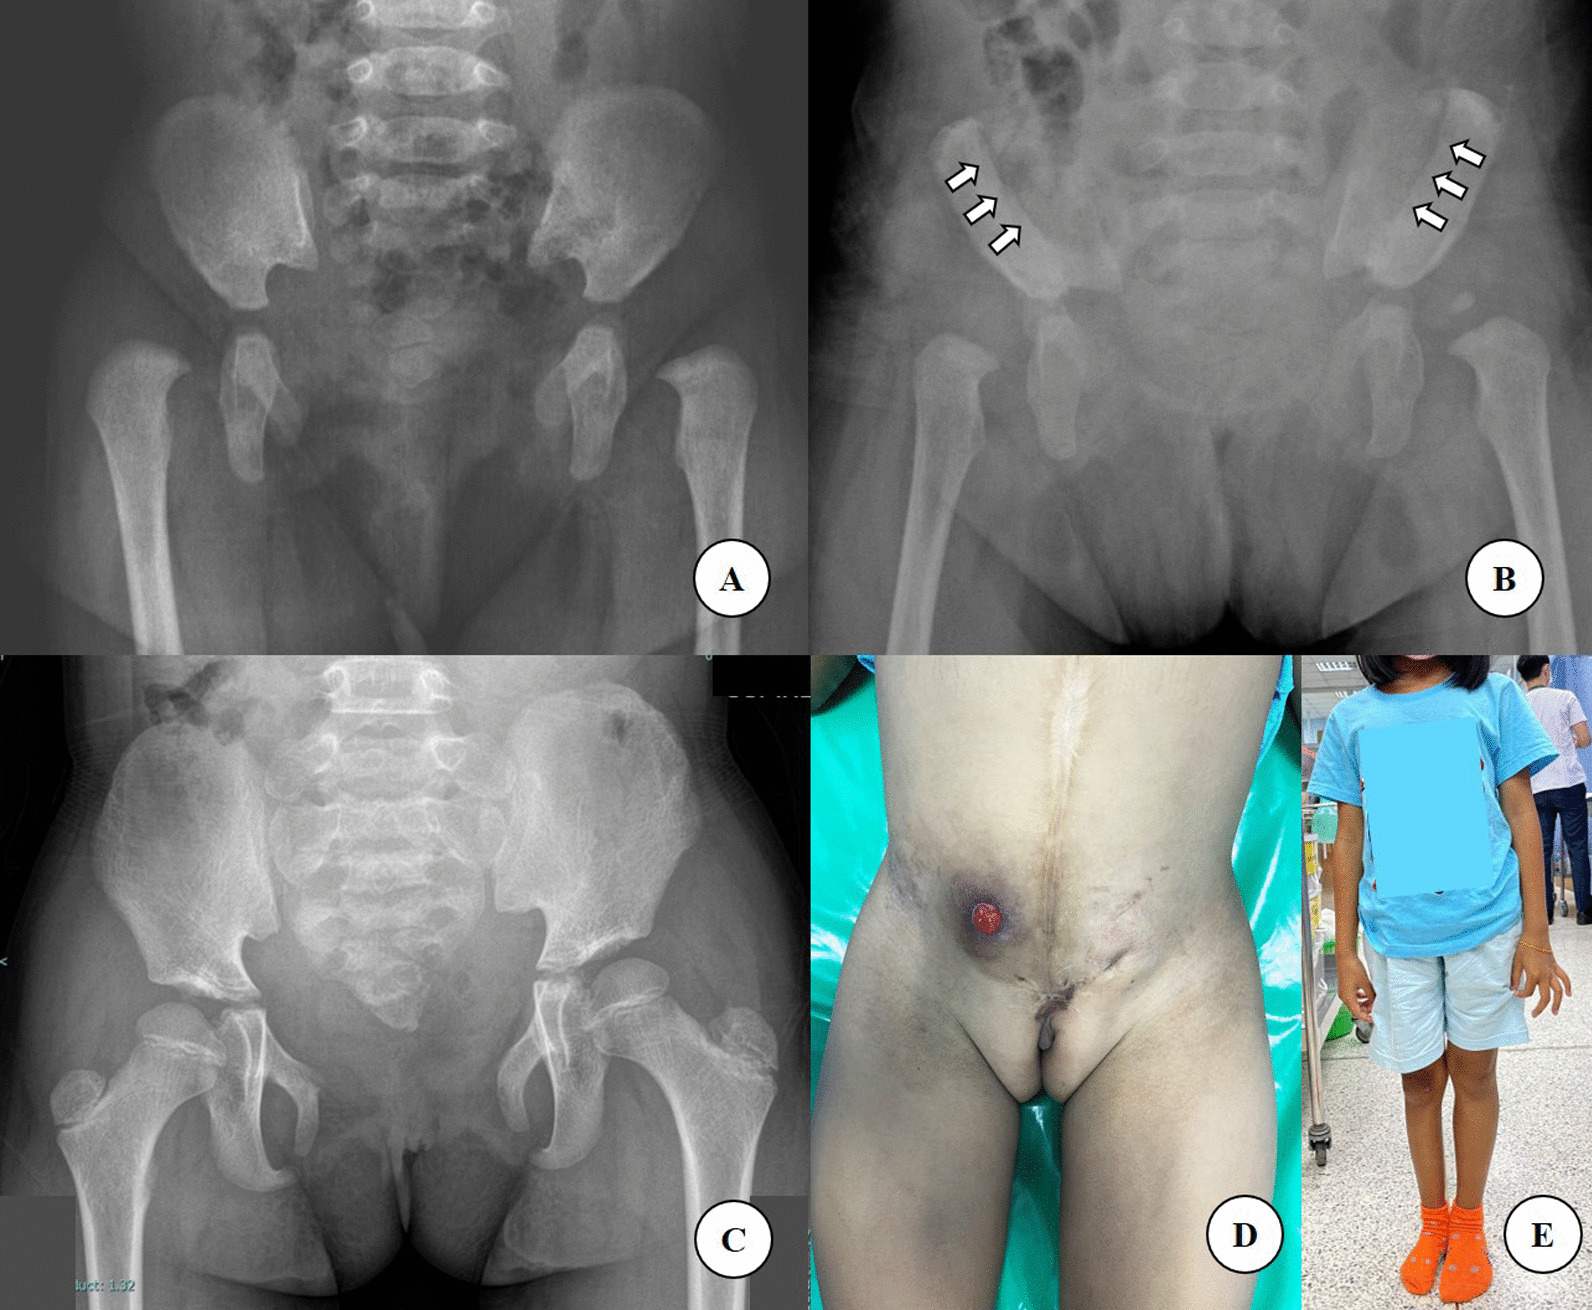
A–E At 6-month-old radiographs of preoperative (A) and postoperative (white arrows emphasized osteotomy sites) (B), and at 9 years follow-up (C) after underwent bilateral bayonet osteotomy and pubic symphysis approximation with Ethibond. Patient presented with successful bladder closure without any clinical symptoms at 9 years follow-up (D, E)
